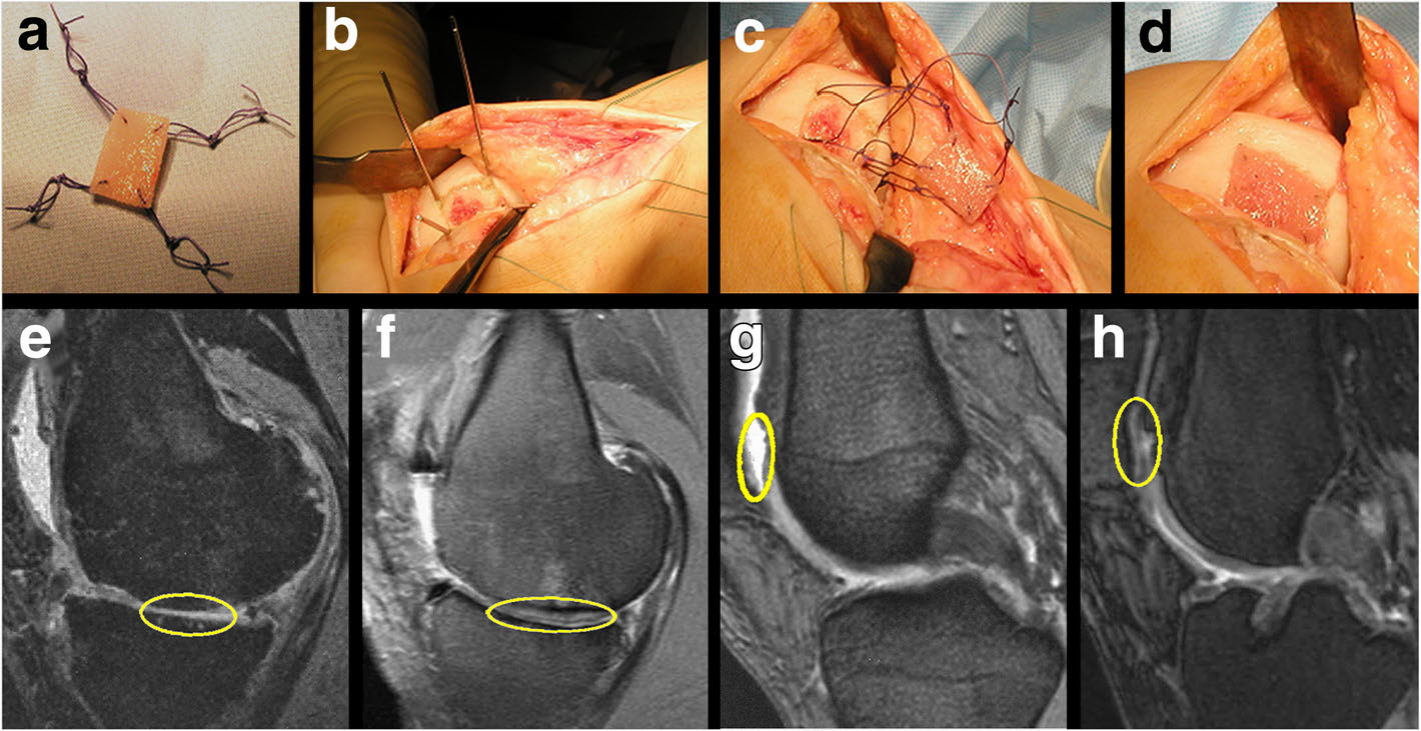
For implantation, BioSeed-C was armed from the corners with resorbable sutures secured by threefold knots (a), k-wires were drilled in the corner of the defect (b), using the k-wires, the guiding threads were pulled through the femoral bone, and the knots were guided into the subchondral bone (c), the knots functioned as anchors, seized the subchondral bone and fixed the implant (d), MRI showing the cartilage defect at the medial femoral condyle before implantation (e) and after four years where the defect was completely filled (f), and the MRI shows a patellar cartilage defect before implantation (g) and after four years where defect was completely filled (h). Adapted with a minor modification from (Kreuz et al. 2009)
